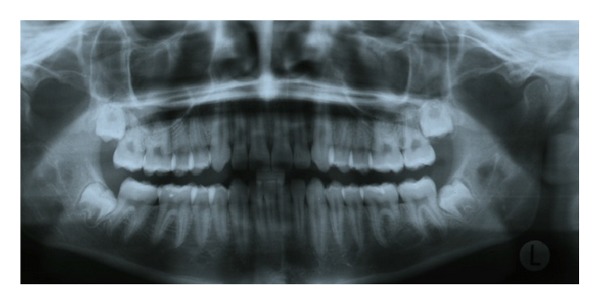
Six-month panoramic radiograph demonstrating no recurrence of lesion, with nascent bone formation.
